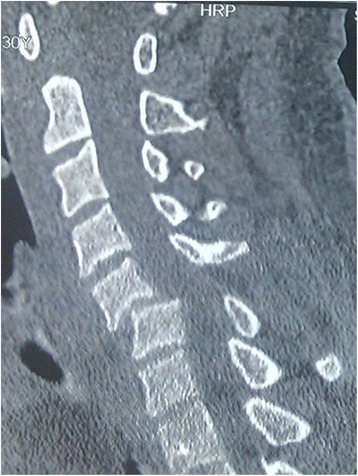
Computed tomography scan before treatment: approximately 50% anterior spondylolisthesis of C5 on the C6 body
